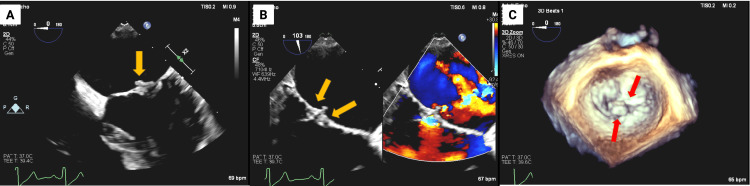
Transesophageal echocardiography (TEE) images. The TEE was the initial cardiac imaging test performed after the diagnosis of multifocal strokes was made. Panel A demonstrates the appearance that led to the initial reporting of flail mitral valve appearance (yellow arrow). Thickened mitral valve leaflet tips (both anterior and posterior leaflets), with kissing lesion appearance (yellow arrows), are shown in panel B, raising suspicion for NBTE and inflammatory etiology. Panel C shows a 3D reconstruction of the mitral valve, with thickening of both mitral leaflet tips (red arrows). NBTE: non-bacterial thrombotic endocarditis
